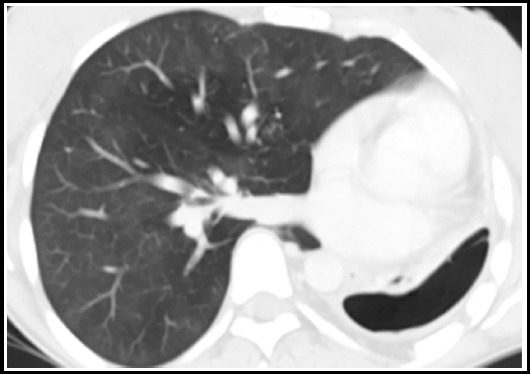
CT scan section in lung window settings, shows loculated left pneumothorax with loss of lung volume on the left. There is compensatory hypertrophy of the right lung field.
